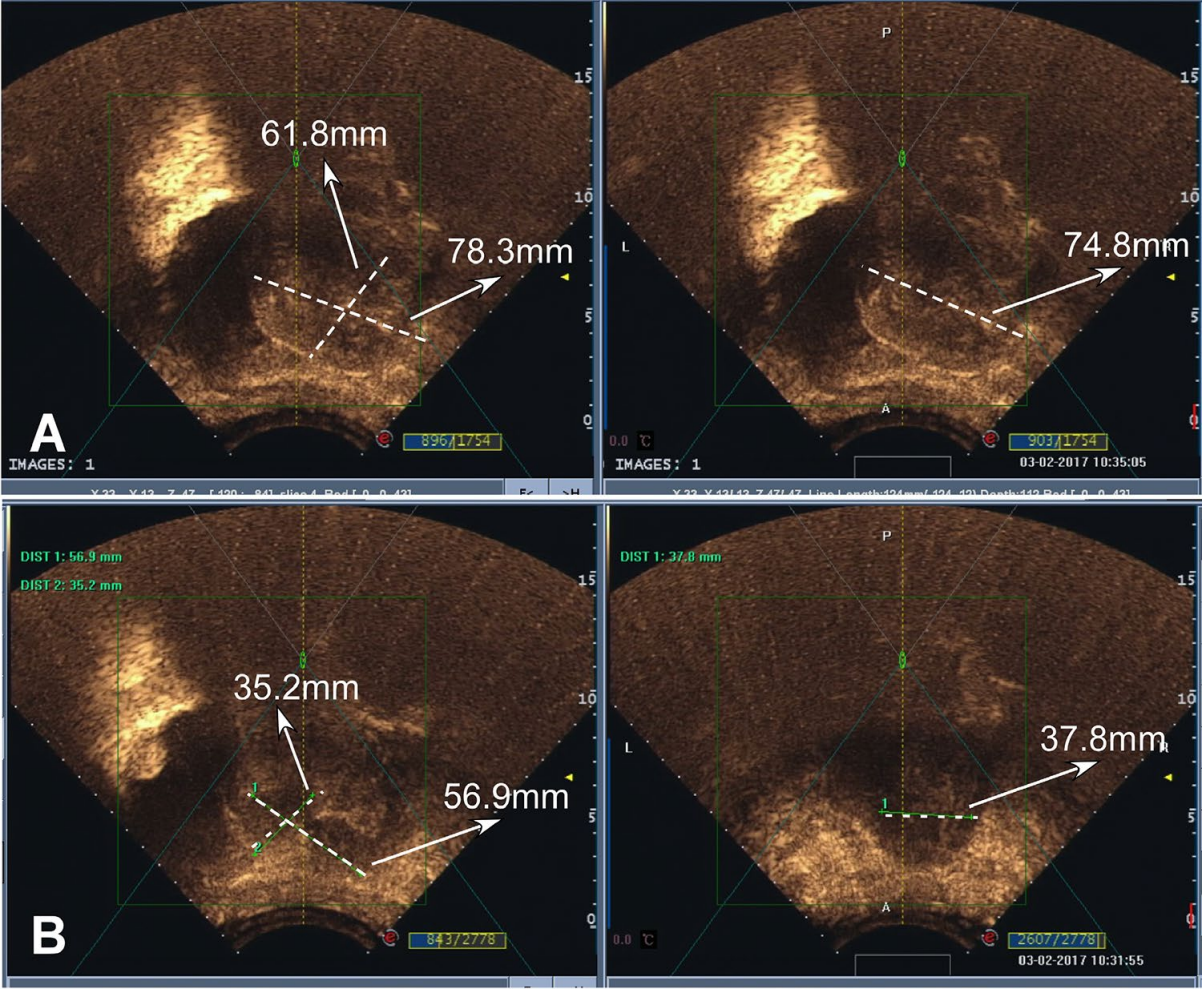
A woman in their 30 s had dysmenorrhea and increased menstrual volume and was found to have uterine adenomyosis. (A) Before high-intensity focused ultrasound (HIFU) treatment, the lesion measured 61.8 mm × 78.3 mm × 74.8 mm on ultrasound imaging. (B) After HIFU treatment, the lesion was reduced to 35.2 mm × 56.9 mm × 37.8 mm.
